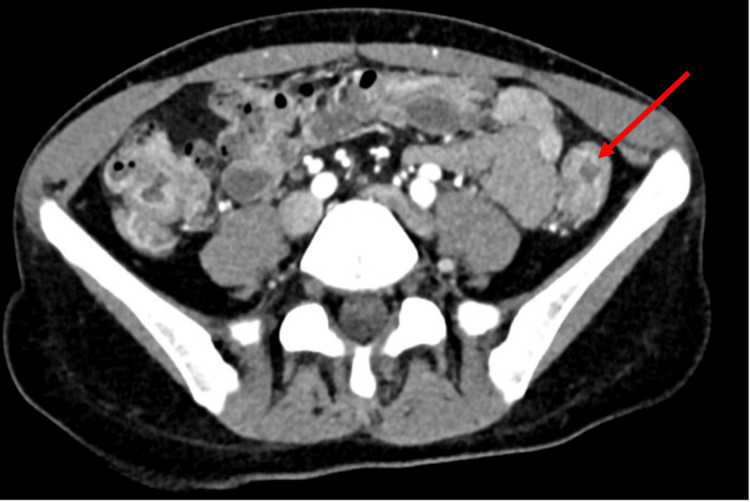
Computed tomography (CT) of the abdomen (axial), obtained with the administration of intravenous contrast material showing multifocal hyperemia and thickening of the colon, predominantly within the distal descending sigmoid (arrow). No signs of obstruction, perforation, or intra-abdominal fluid accumulation are present.
